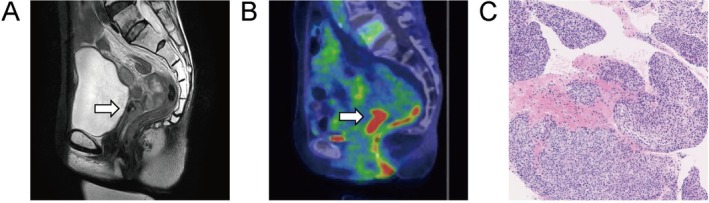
Findings for recurrence. (A) MRI (T2‐weighted image) showed a recurrent tumor of about 2 cm at the vaginal cuff after hysterectomy (arrow). (B) PET‐CT showed uptake in the tumor at the same location of the vaginal cuff identified on MRI (arrow). (C) Pathological findings from a transvaginal tumor biopsy indicated squamous cell carcinoma, similar to the primary lesion.
